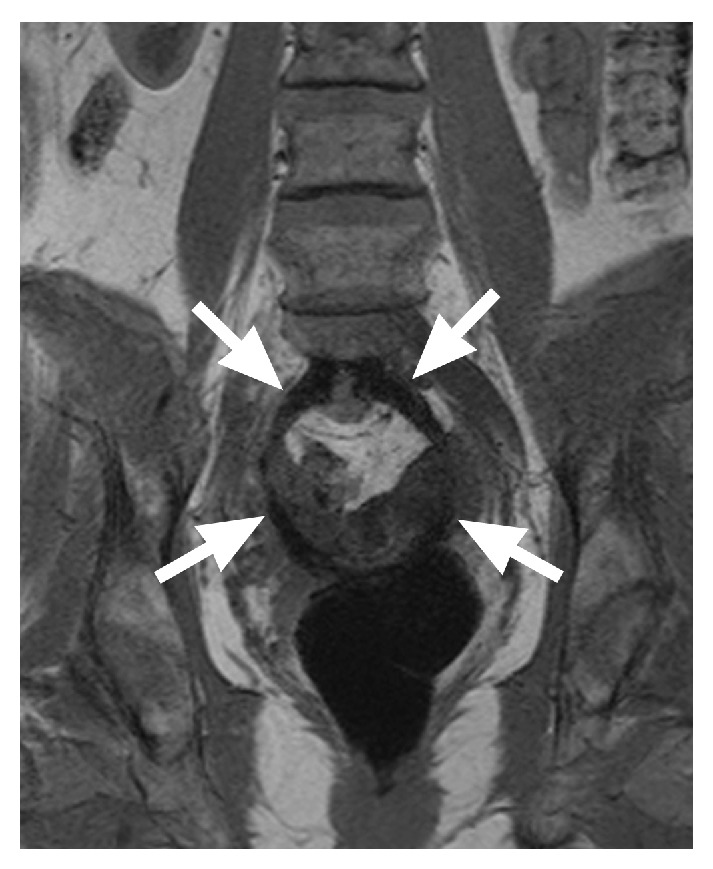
Same patient as in Figure 6, this time a T1-weighted dataset, again with a coronal subvolume minimum intensity projection. Using the T1-weighted images, the contrast between the implant and the surrounding tissue is even better. Due to the iron oxide particles, a signal loss in the area of the implant is obvious (arrows), which allows for exact identification of the implant.
